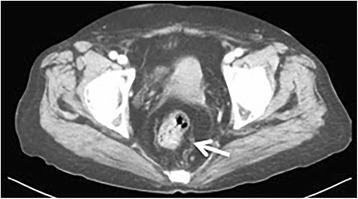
Pelvic contrast computed tomography (CT) scan showing wall thickening with contrast effect in the rectum and swelling of the lymph nodes
